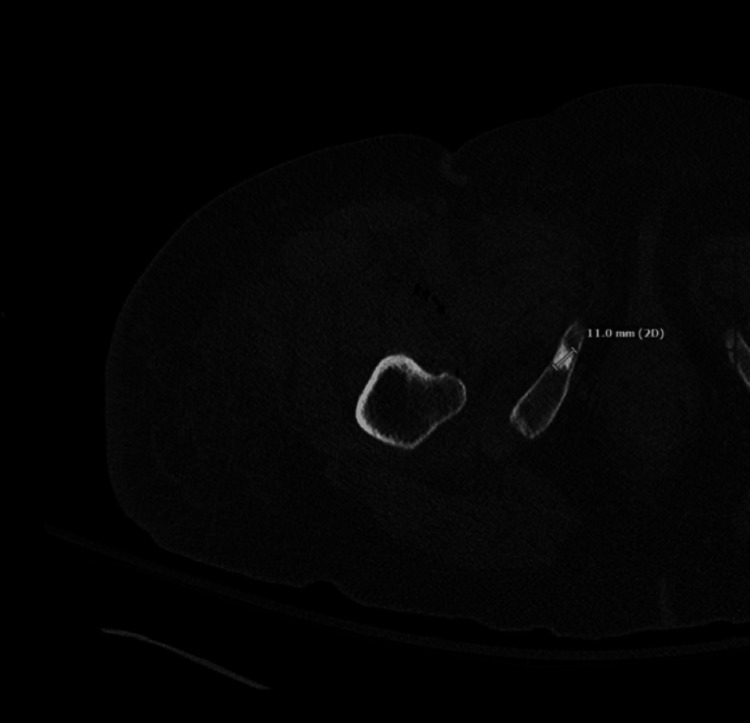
CT of the right lower extremity without contrast showing inflammatory changes with soft-tissue air tracking from the right iliopsoas musculature to the right mid-quadriceps musculature. No drainable, well-defined fluid collection was identified.
